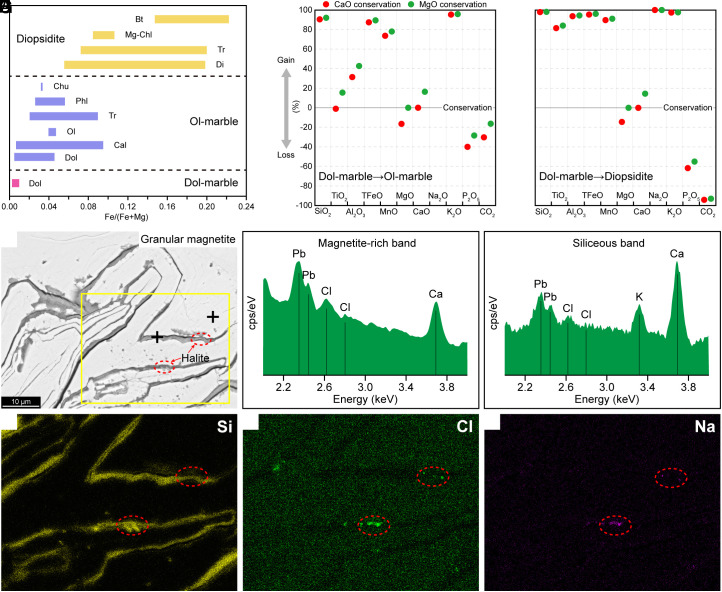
(*A*) Fe/(Fe + Mg) ratios of minerals from dolomitic marble, olivine marble, and diopsidite. (*B*) Mass balance calculations on the basis of CaO or MgO conservation showing mass gain and loss of components in olivine marble. (*C*) Mass balance calculations on the basis of CaO or MgO conservation showing mass gain and loss of components in diopsidite. (*D*) BSE image showing granular magnetite. (*E* and *F*) EDS analyses showing trace chlorine in magnetite-rich and siliceous bands. Analytical points are marked with plus symbols in image (*D*). (*G*–*I*) X-ray maps of Si, Cl, and Na showing halite grains in siliceous bands. The mapping area is marked with a yellow rectangle in image (*D*).
